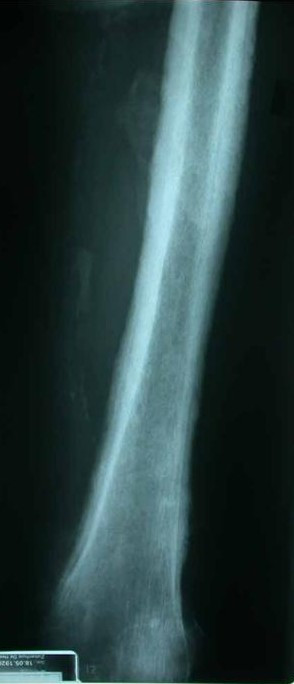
X-ray of the femur showing extensive characteristic periosteal bone apposition.
